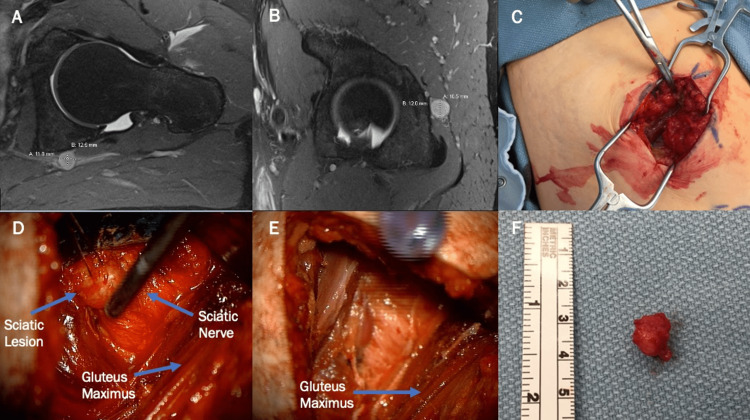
Case 1 preoperative and intraoperative imaging The patient had a 1.1x1.2x1.1cm left sciatic homogenous lesion off the sciatic nerve as it exits the sciatic notch as seen on the axial (A) and sagittal (B) T1 contrast MRI. A minimally invasive linear incision over the lesion (C) was used to use a transgluteal approach to the sciatic notch revealing the lesion (D & E). In addition, an en bloc resection was achieved (F).
